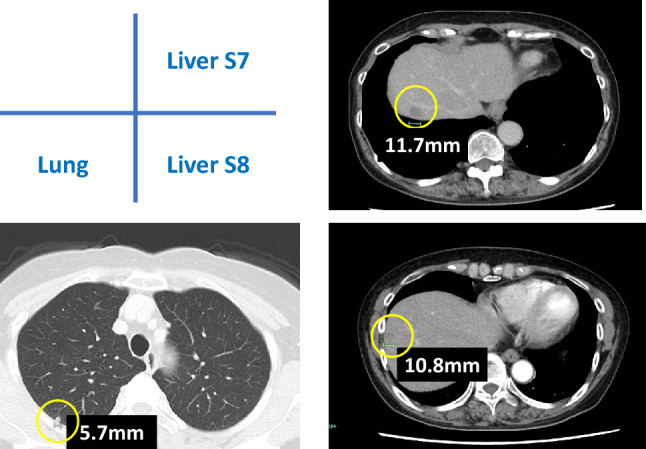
Computed tomography image before initial liver resection
